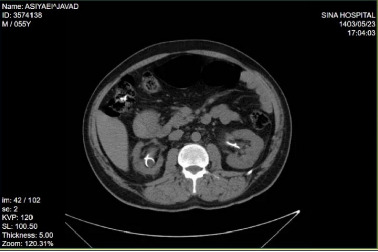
Noncontrast CT of abdominal pelvic with bilateral double J after surgery.
